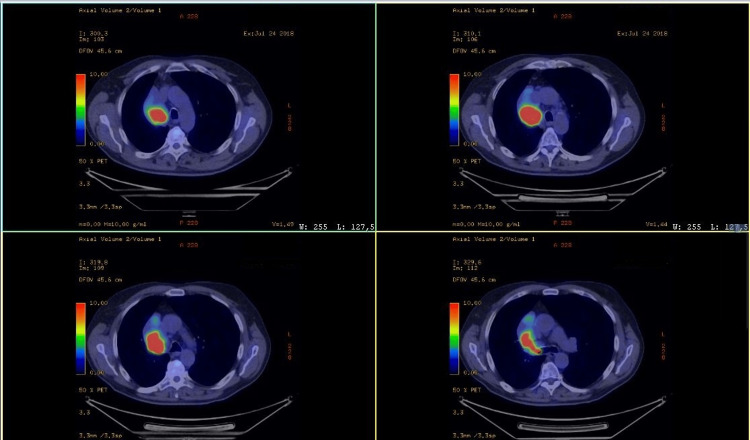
Initial PET CT scan thorax PET: Positron emission tomography
